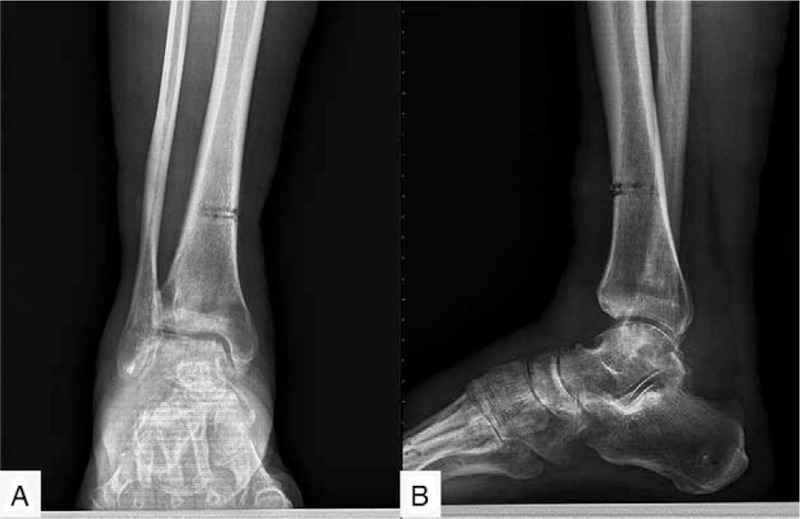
The weight-bearing radiograph showed that the joint space remained good after the external fixator was removed.
